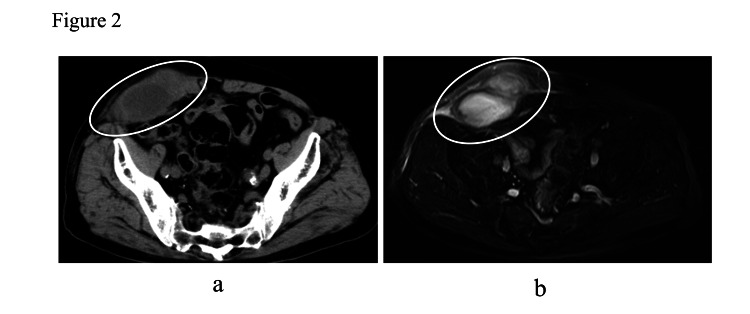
Second primary abdominal wall abscess (A) Computed tomography revealed a 70 × 40 mm low-density mass at the lower abdominal wall, distant from the first one. The mass also had no connection with any intraperitoneal organs or lymph nodes. (B) Magnetic resonance imaging findings.
